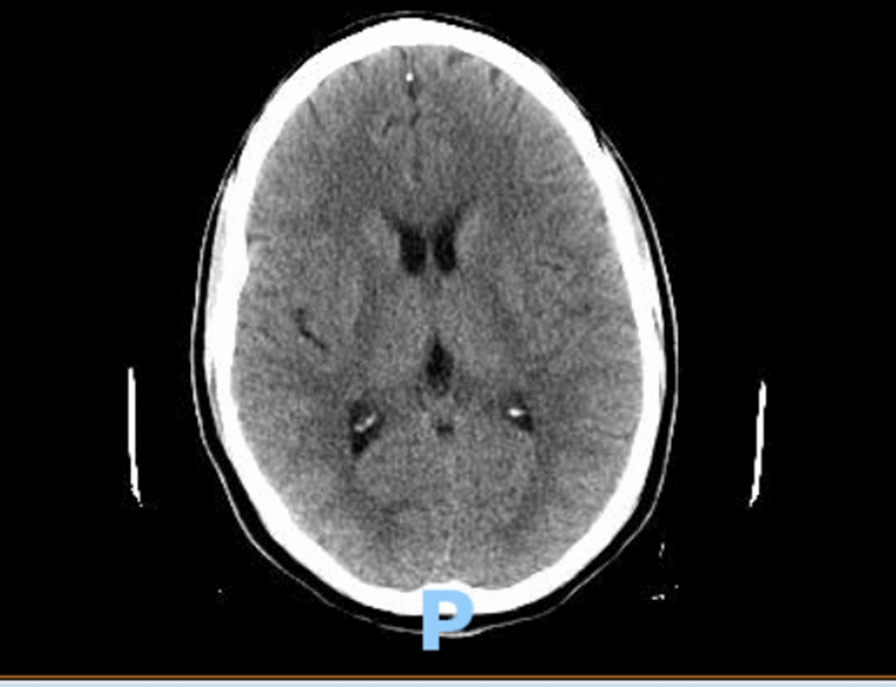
CT head without any evidence of intracranial changes, cerebral edema, mass effect, or midline changes
